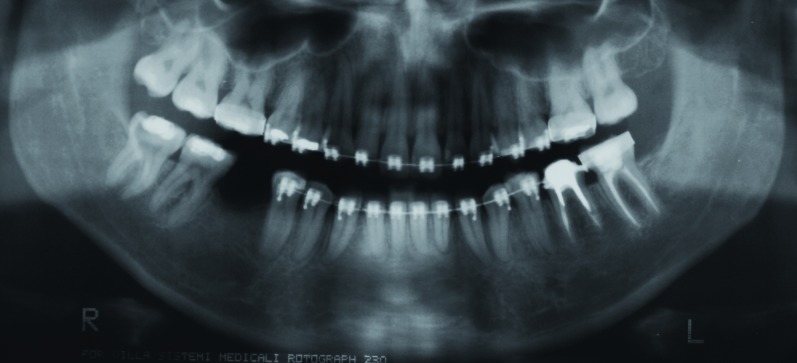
Panoramic radiograph showing total regression, one year after the incisional biopsy.
